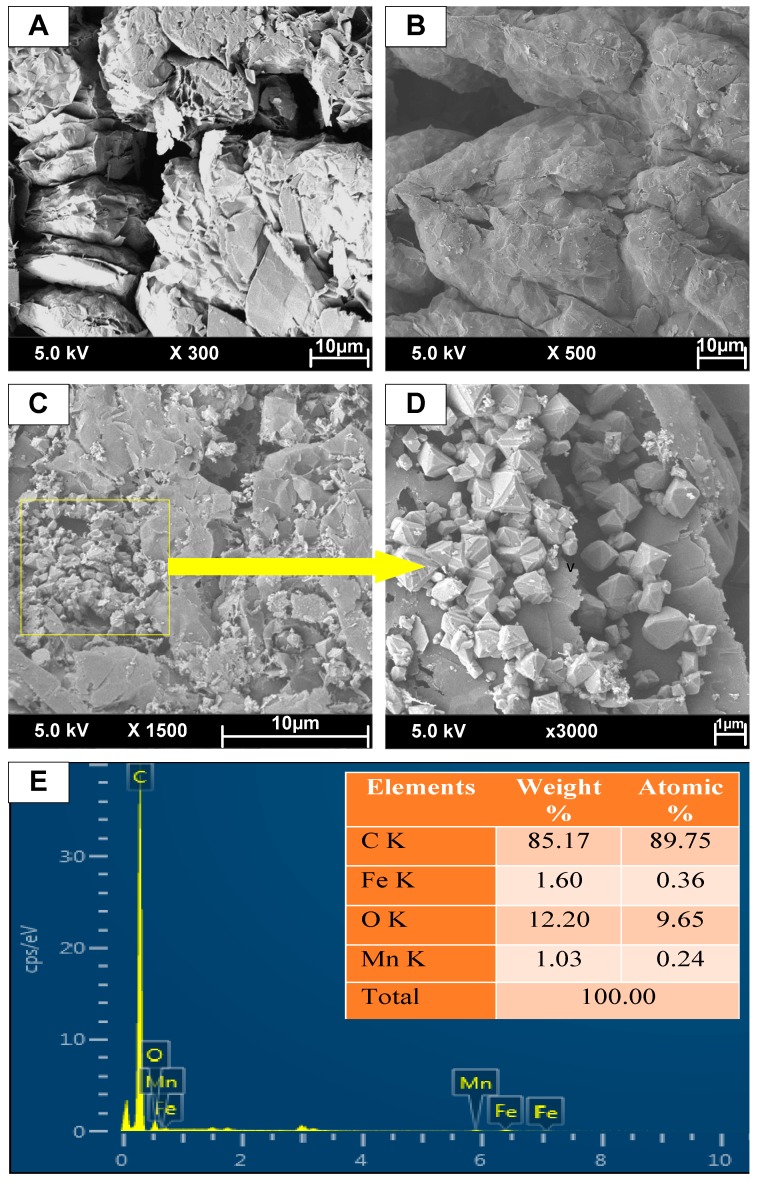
SEM images of expanded graphite (EG) (A), EG-MnFe_2_O_4_ (B), details of EG-MnFe_2_O_4_ (C,D), and Energy-Dispersive X-ray (EDS) of EG-MnFe_2_O_4_ (E).
